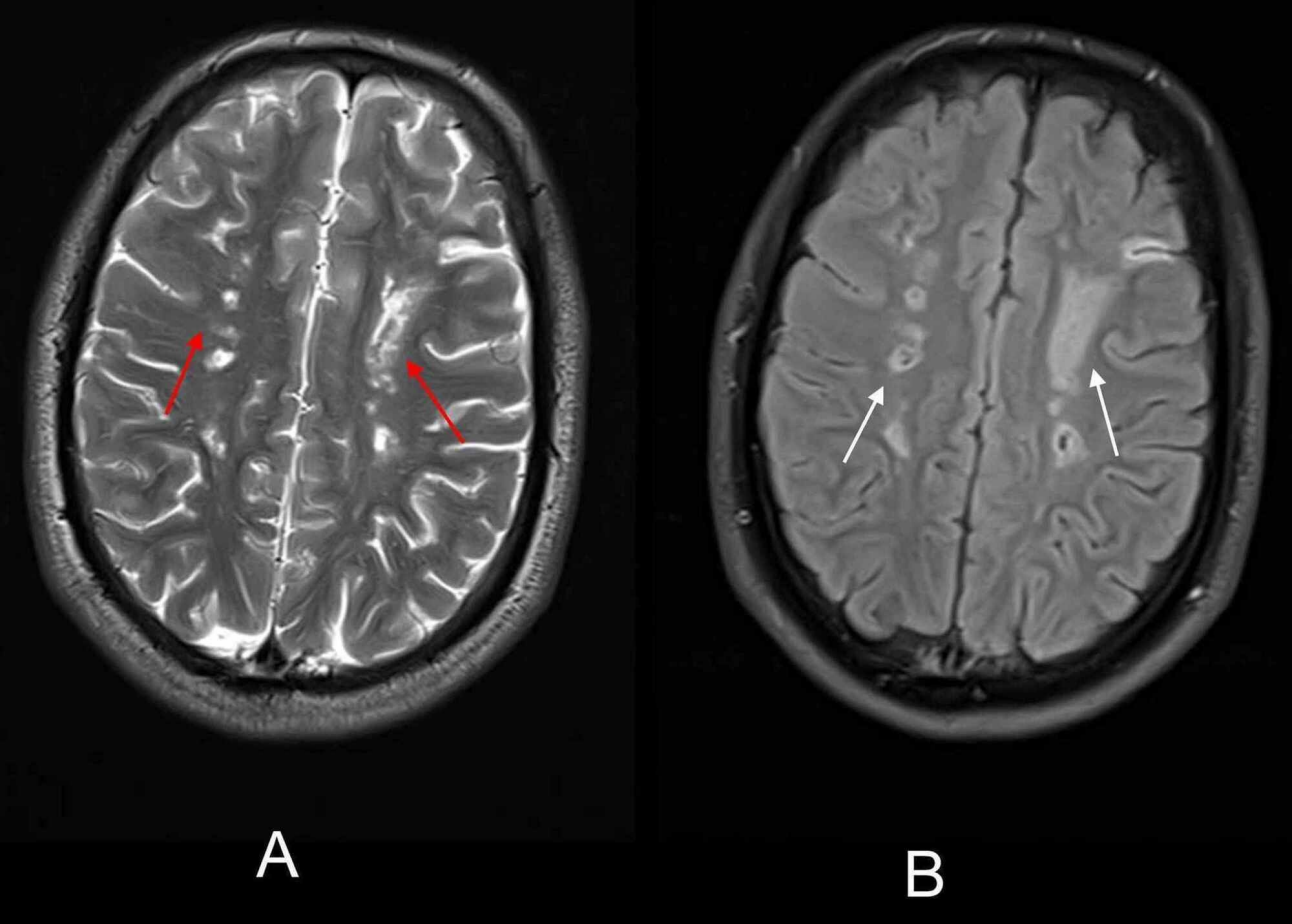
Axial T2-weighted and FLAIR sequence of the second brain MRI showing extensive chronic ischemic infarcts Red arrows on the axial T2-weighted image (A) and white arrows on FLAIR image (B) show multiple chronic ischemic infarcts in bilateral centrum semi-ovale and frontoparietal lobes. FLAIR - fluid-attenuated-inversion recovery
